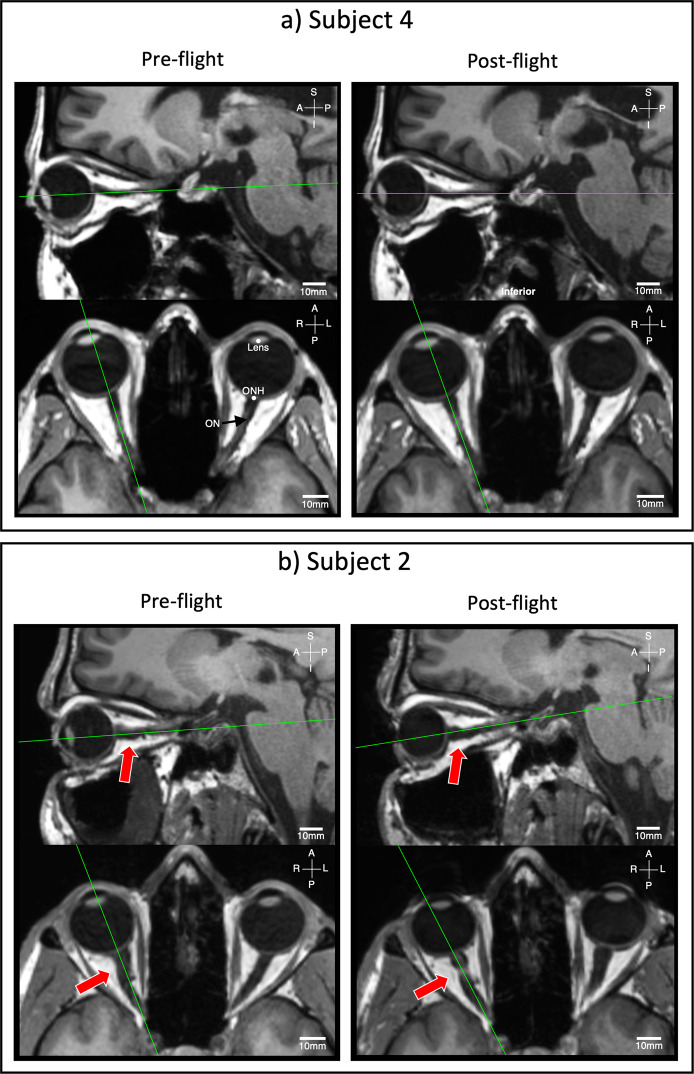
Sample MRIs used for ON deviation quantification. Preflight and R+1 T1-weighted sagittal MRI of 2 astronauts showing astronauts a without and b with ON deviation after spaceflight in both sagittal (top row) and axial (bottom row) views. Red arrows highlight the largest ON deviation in our cohort and green lines identify the locations of the orthogonal images. S–I superior and inferior, A–P anterior and posterior, R–L right and left.
